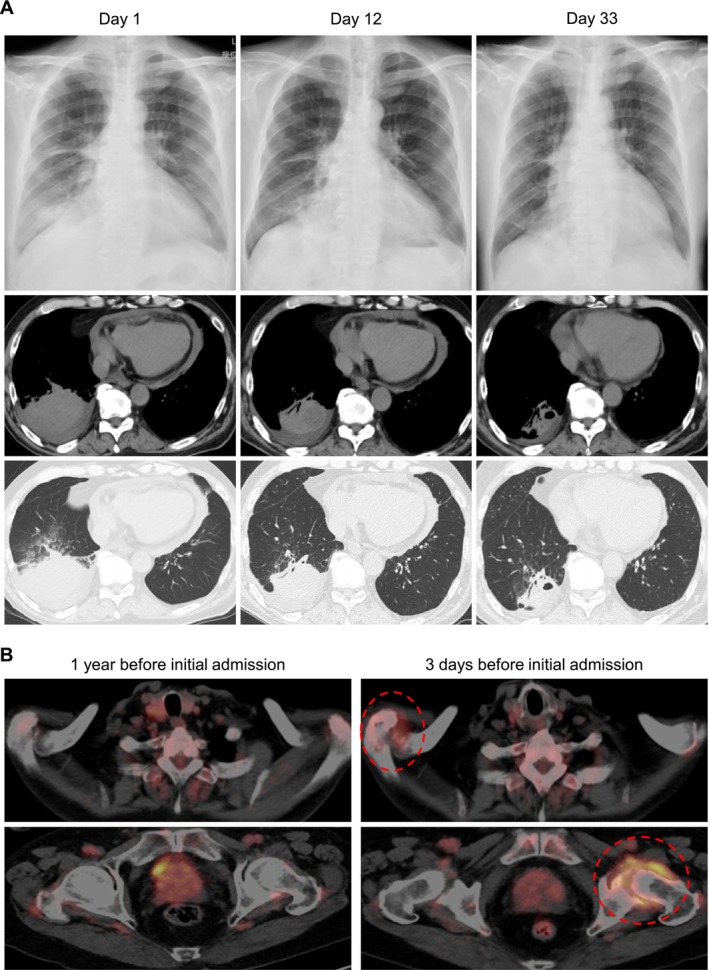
Serial radiological findings and FDG‐PET/CT images. (A) Chest radiographs obtained on Day 1, Day 12 and Day 33 demonstrate gradual improvement of right lower lung opacity. Axial chest CT images show consolidation with pleural effusion in the right lower lobe on Day 1, consistent with lung abscess formation, followed by progressive reduction on Day 12 and Day 33. Corresponding lung window images reveal residual organizing changes during the recovery phase. (B) FDG‐PET/CT images obtained 1 year before admission and 3 days before initial admission demonstrate newly developed focal fluorodeoxyglucose uptake in the right shoulder and left hip joints (red dashed circles), findings that were absent on the previous study and are suggestive of preclinical inflammatory arthritis. CT, computed tomography; FDG, fluorodeoxyglucose; PET, positron emission tomography.
